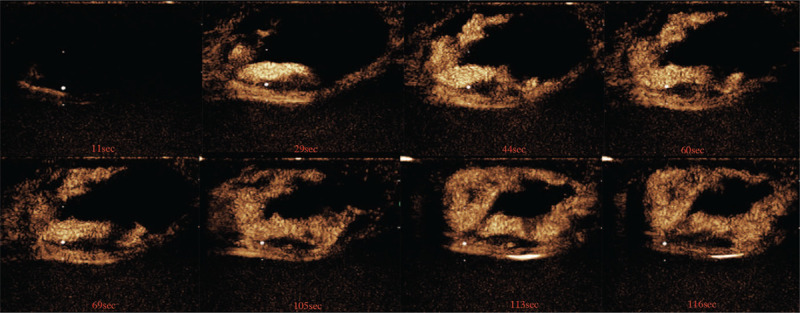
Contrast-enhanced ultrasound images of the left breast lesion after injection of a SonoVue.
